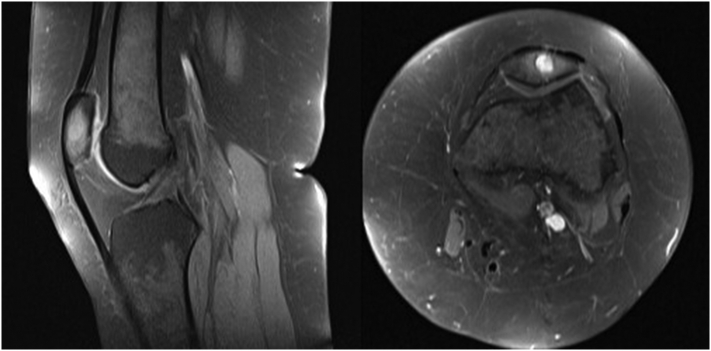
MRI T1-weighted with fat saturation coronal and axial sections showing hyperintense well defined lesion in the left patellar.
